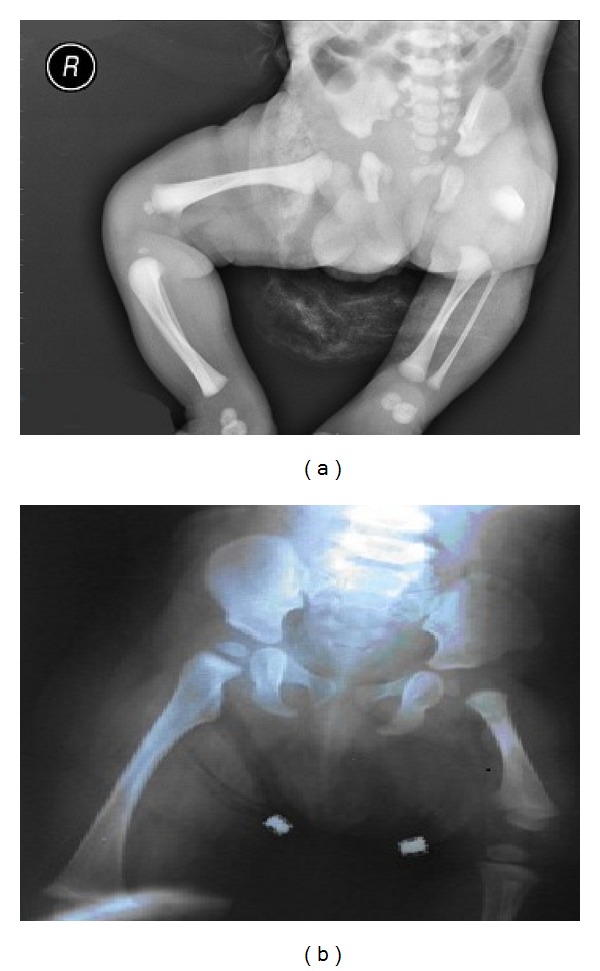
The first week after birth (a) and 18 months later (b) with plain radiography, comparative view of the left and right femurs. Normal right lower extremity and left femoral deficiency in (a). The shortening of left femur, femur head and neck in (b).
